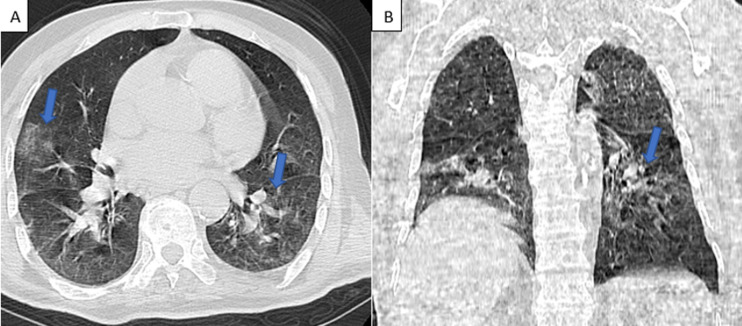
thoracic CT scan in the axial: A): and coronal plane; B): revealed 30% of CT abnormalities related to the COVID-19 infection
